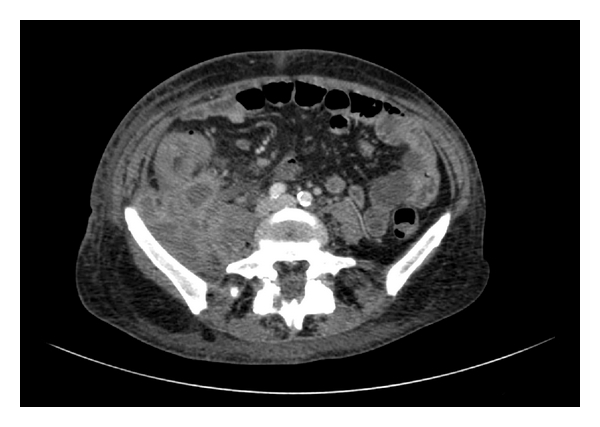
Computed tomography (CT) scan of the abdomen showed the mass occupying the retroperitoneal space and infiltrating the ascending colon.
